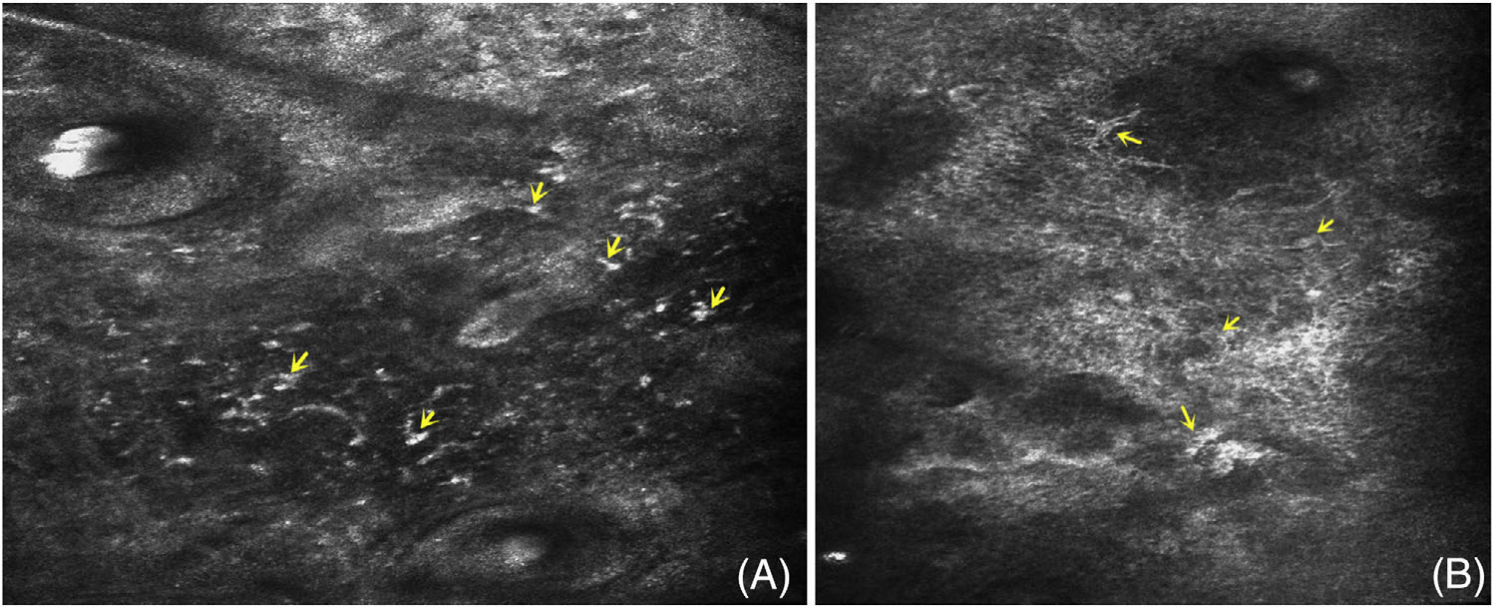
(A) The CT image of rosacea skin shows that there are many large high refraction cell particles infiltrating around the hair follicle and the shallow and middle layer of dermis (yellow arrow); (B) dendritic cells (yellow arrow) can be seen in some rosacea.
